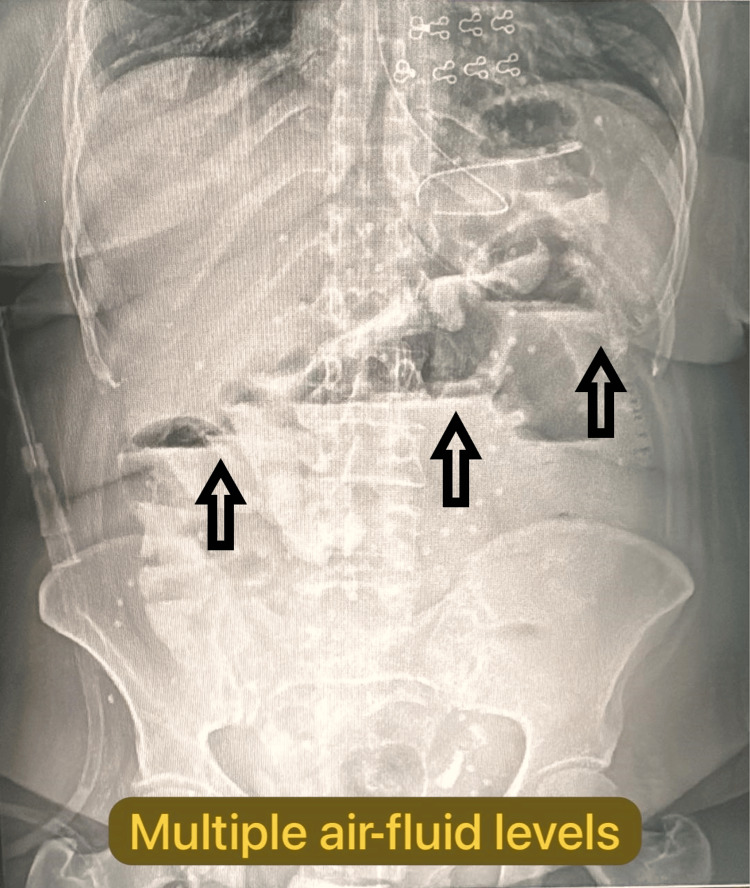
A plain abdominal radiograph demonstrating multiple dilated small bowel loops with air-fluid levels, consistent with proximal small bowel obstruction
